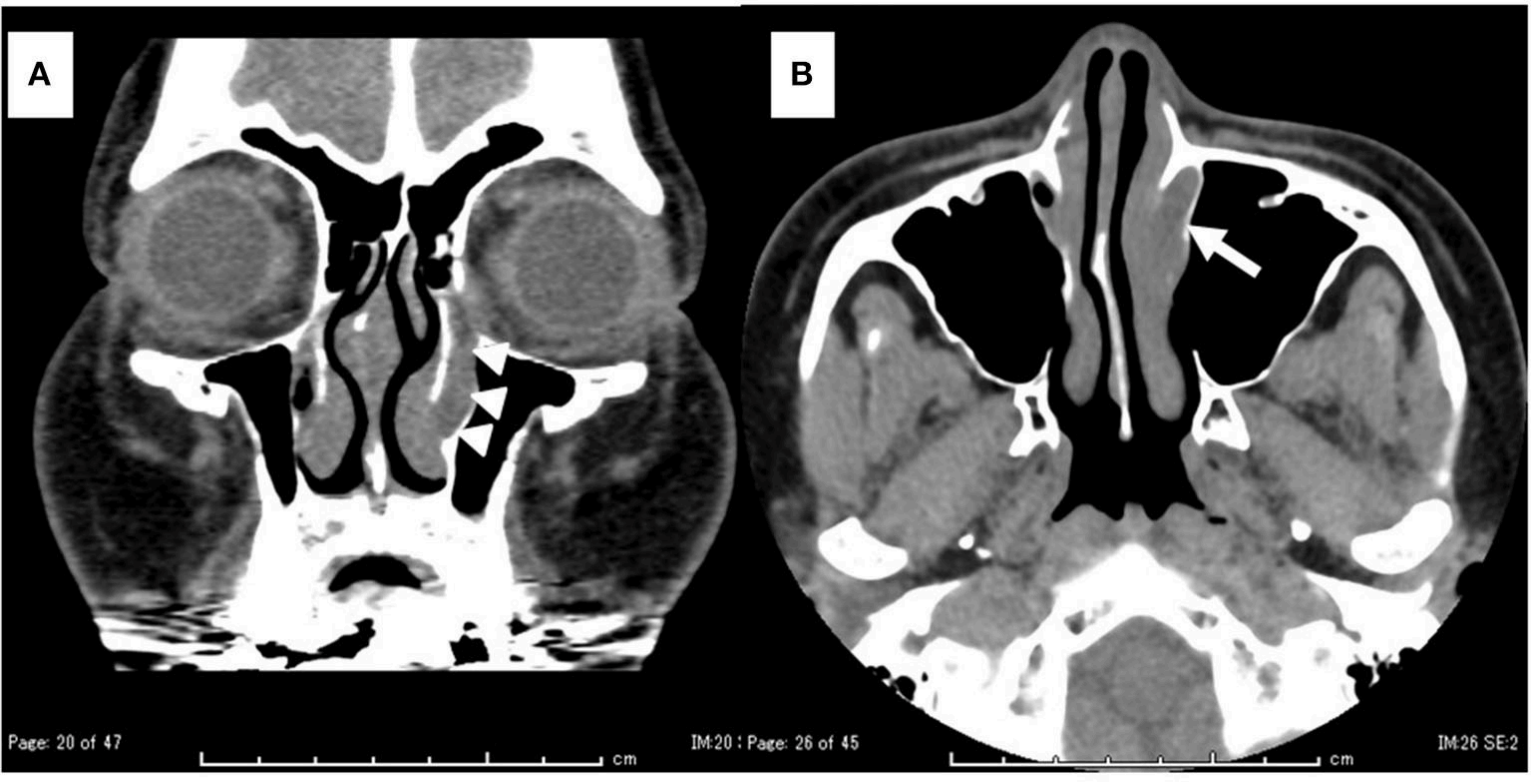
Computed tomography (CT) findings. (A) Coronal view: the left nasolacrimal duct was enlarged from the lacrimal sac to the orifice (white arrowhead). (B) Axial view: the left nasolacrimal orifice was enlarged (white arrow).
